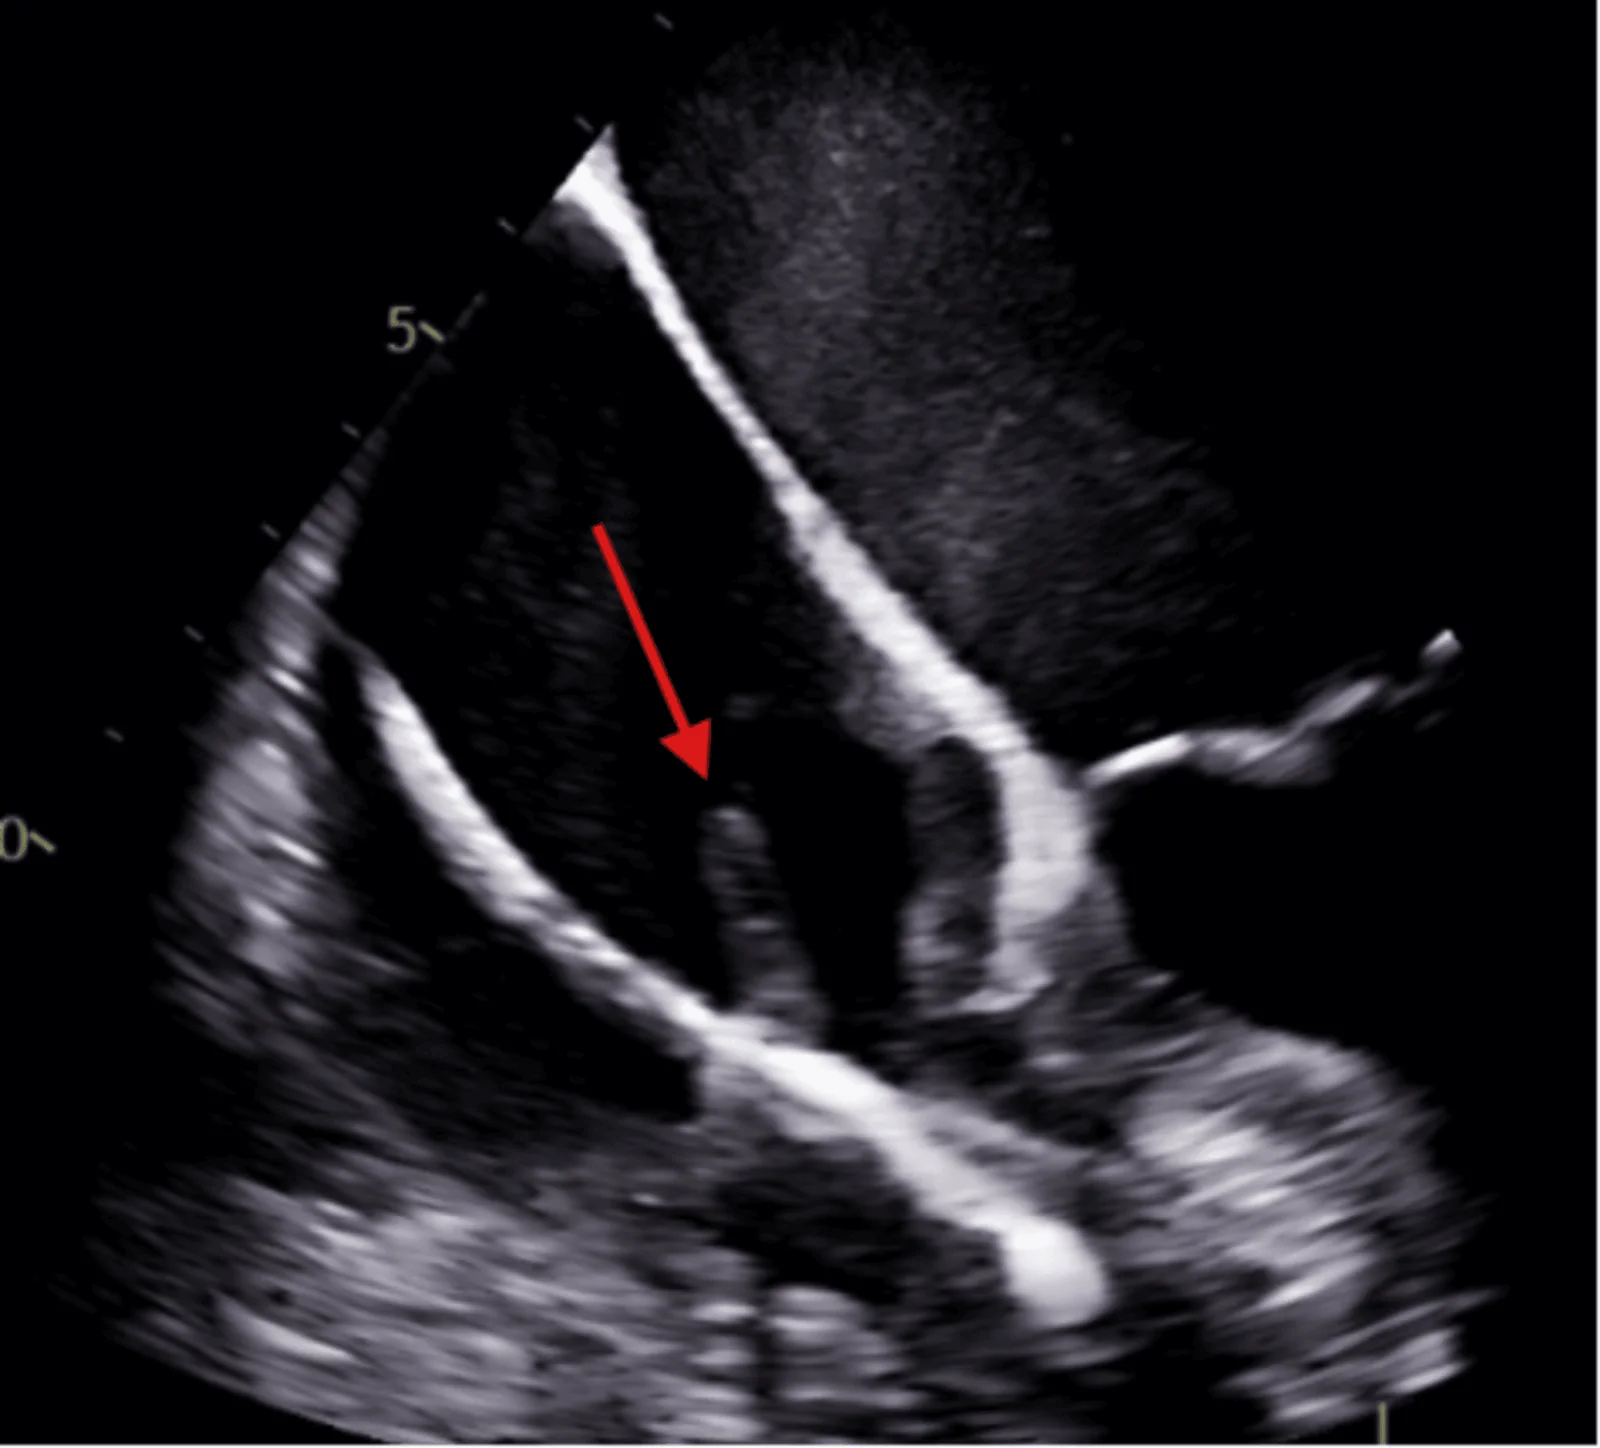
CRT-D lead vegetation. TEE image showing a mobile echodensity attached to the CRT-D lead (arrow), consistent with lead-associated vegetation in the setting of *Streptococcus agalactiae *bacteremia. CRT-D, cardiac resynchronization therapy defibrillator; TEE, transesophageal echocardiography
